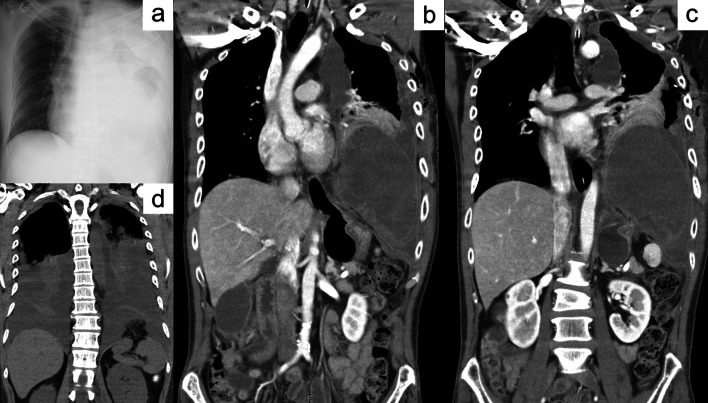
Preoperative imaging findings. a Chest X-ray showing the opacity of the entire left lung field with pleural fluid and left intrathoracic free air. The trachea and mediastinum shifted to the right. b Coronal images of the enhanced computed tomography (CT) scan on admission revealed that the fundus and corpus of the stomach with reduced contrast enhancement herniated to the left thoracic cavity through a defect in the diaphragm. The cardia and pylorus of the stomach presented normal enhancement. c The left lung is excluded by the gastric content of the herniated body, intrathoracic free air, and pleural effusion. d A plain CT scan obtained during the patient’s previous admission showed a small defect in the left hemidiaphragm with a herniated omentum
